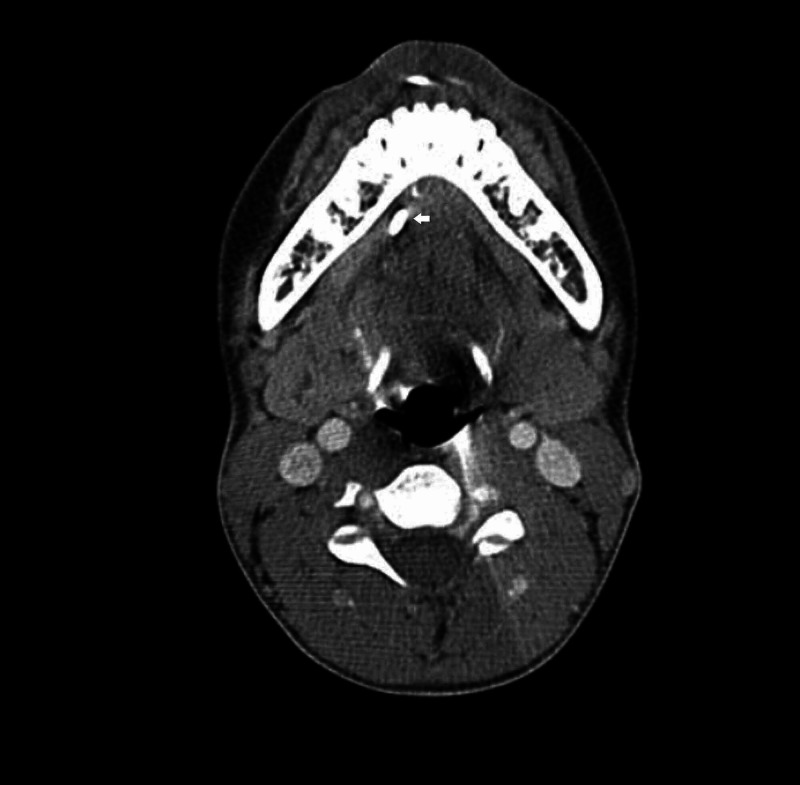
CT scan of the neck (axial view) with a stone (white arrow) seen in the Wharton's duct
